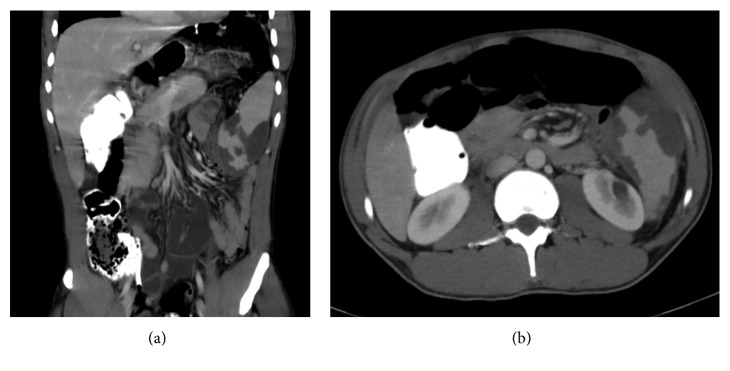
Coronal (a) and transverse (b) sections of the abdominal computed tomography scan showing multiple subcapsular hematoma in the spleen.
